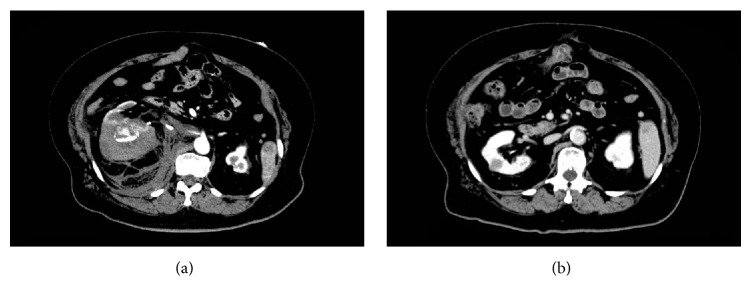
Computed tomography (CT) findings. (a) The initial enhanced CT (arterial phase), depicting massive retroperitoneal bleeding. (b) The previous CT (late portal phase), performed one year before the emergent admission, depicting the renal tumor mimicking a simple renal cyst.
